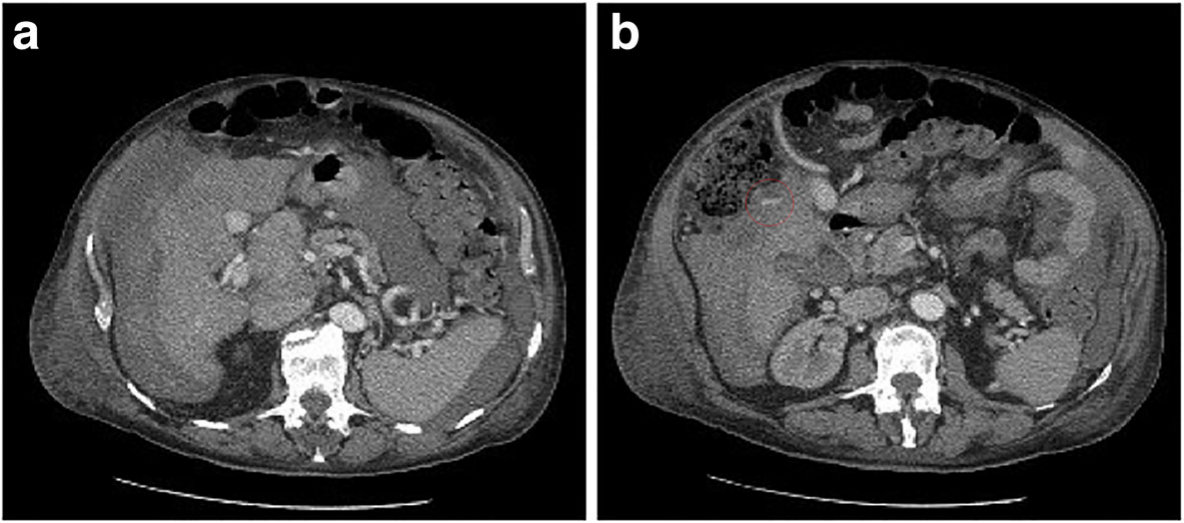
Abdominal computed tomography scan with intraperitoneal fluid with blood density (a), and evidence of the contrast leak (*white spread*) in proximity of the right lobe of the liver (b)
